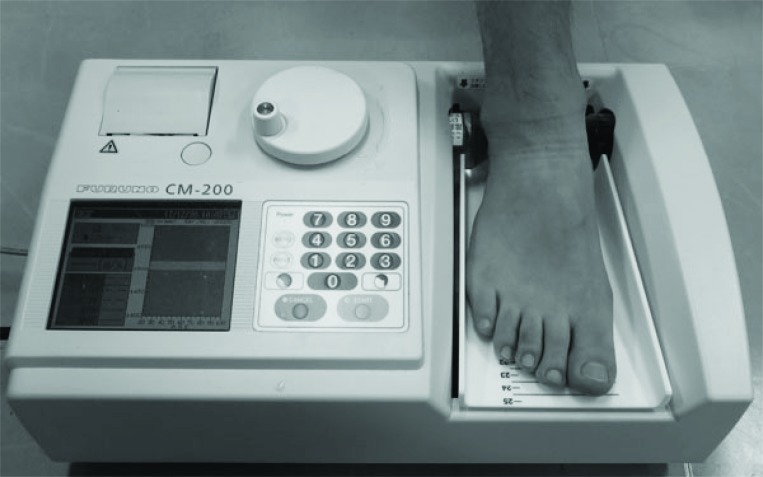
Measurements of quantitative ultrasound assessment of calcaneus by using CM-200 ^TM^.
